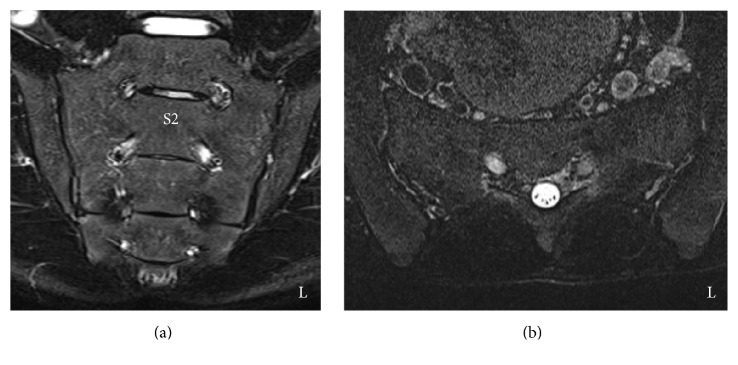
Magnetic resonance images of the sacrum one year after conservative treatment. (a) Coronal plane on a short TI inversion recovery (STIR) image. (b) Axial plane at the S2 level on a STIR image. Coronal plane (a) and axial plane at the S2 level (b) on a STIR image show no abnormal signal intensity. These radiological findings indicated bone union of the sacral stress fracture.
